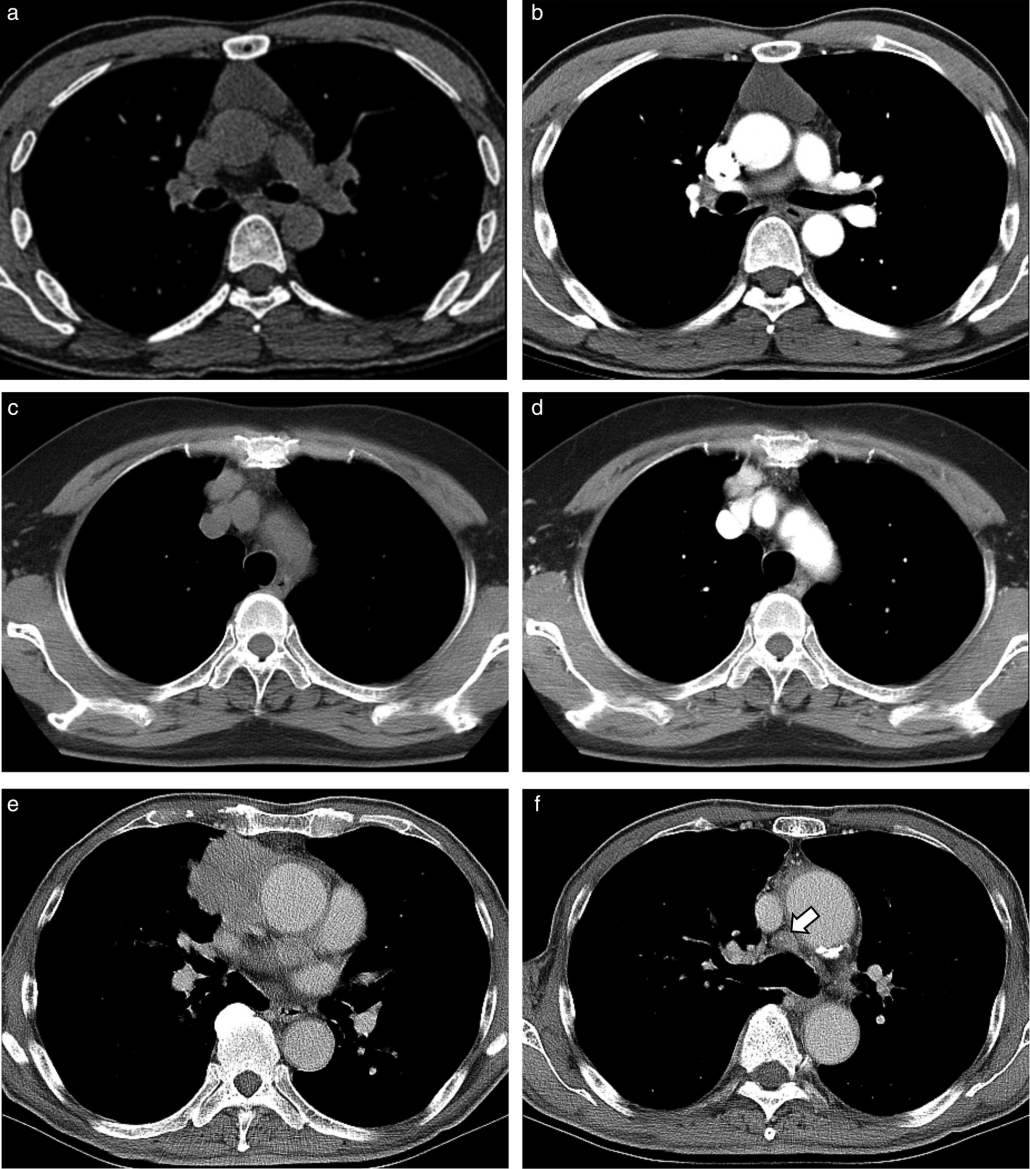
Three representative cases CT scans of three pathologies. (a, b) A 43‐year‐old male patient presented with a 4.3 cm sized unilocular cystic lesion confirmed to be a thymic cyst. The lesion showed smooth contour and well‐demarcated margin without any focal necrotic area, and was located in the median position. The attenuation of the lesion was less than 20 in both pre‐ and postcontrast scans. (c, d) A 67‐year‐old female patient presented with a 2.2 cm sized eccentric‐located lobulated‐contoured lesion without evidence of local invasion or distant metastasis. The lesion showed soft tissue‐attenuation on the precontrast scan (42 HU) and contrast enhancement (151 HU after contrast media injection). The lesion was confirmed to be thymoma after resection. (e, f) A 70‐year‐old male patient showed 10.9 cm sized mass with adjacent lung/venous invasion and mediastinal lymph node enlargement (arrowed). This lesion was surgically confirmed to be thymic carcinoma.
